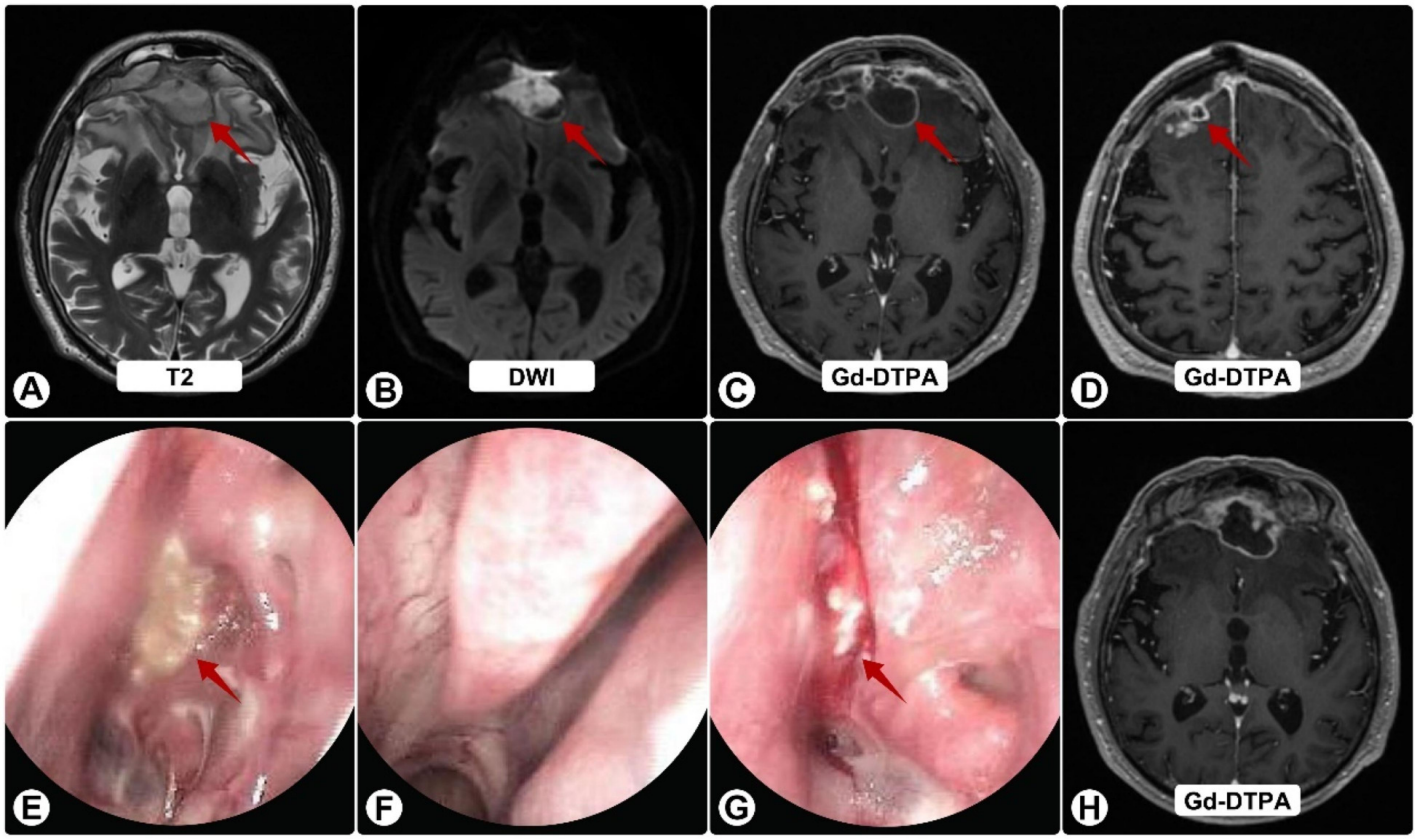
Follow-up brain MRI and nasal endoscopy. (A) T2-weighted image showing enlargement of frontal lesions (red arrows) with increased edema. (B) DWI shows persistent restricted diffusion. (C,D,H) Gd-DTPA–enhanced images demonstrate continued ring enhancement, with some solid components (H), (E–G) Endoscopy reveals persistent purulent secretions (red arrows) on the nasal mucosa (E), left middle meatus (F), and sphenoid sinus ostium (G), despite antibiotics.
